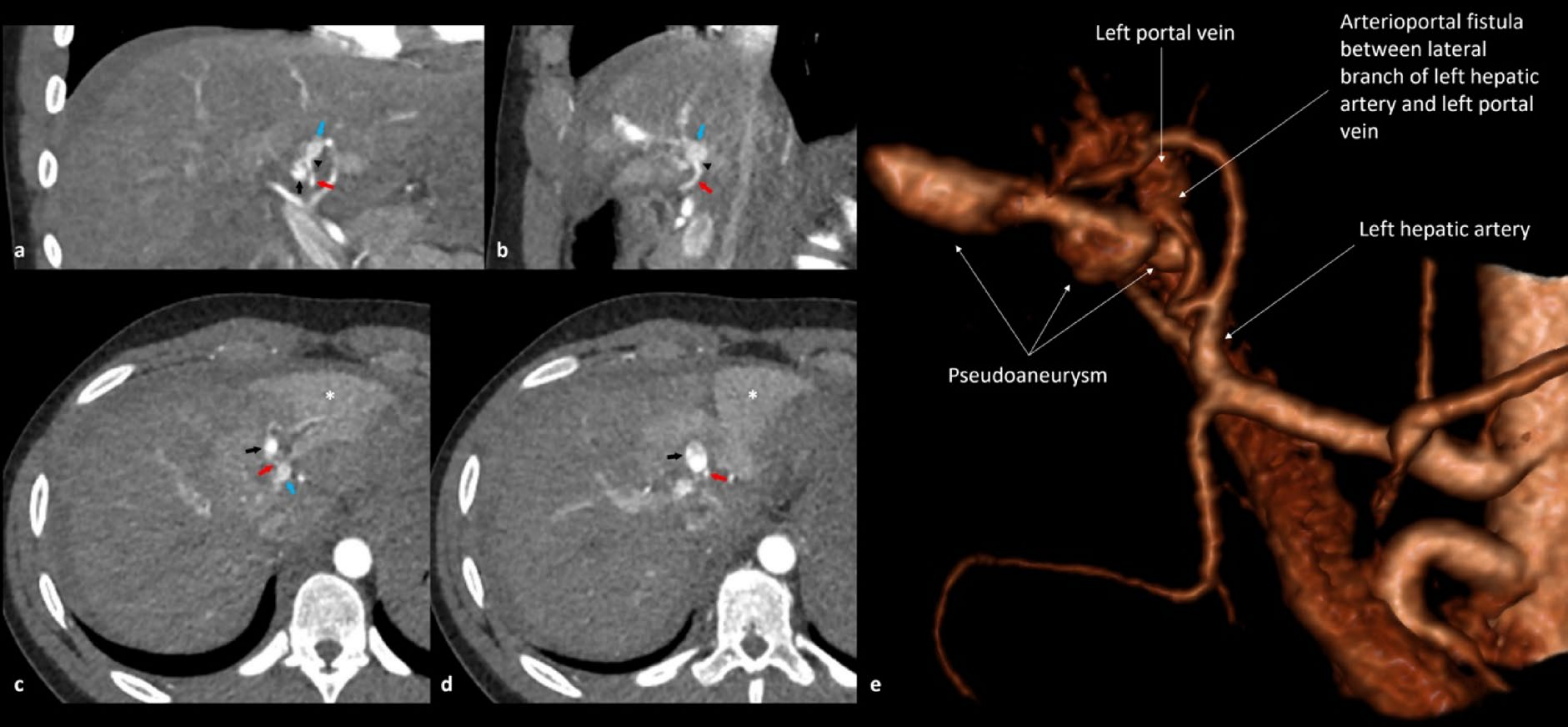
Parasternal right-sided stab wound with injury to the liver. Images a-d show the trauma CT scan in arterial phase in coronal (a), sagittal (b), and axial views (c and d). There is an arterioportal fistula (black arrowhead) between the lateral branch of the left hepatic artery (red arrow) and the left portal vein (blue arrow) with formation of a pseudoaneurysm (black arrow) extending along the stab wound tract in the left liver lobe. Note the arterioportal fistula associated transient hepatic attenuation difference (THAD) of the left liver lobe (white asterisk). e) The image represents the corresponding 3D rendering of the same CT scan. The arterioportal fistula (white arrow), the left portal vein (white arrow), the pseudoaneurysm (white arrows) and the left hepatic artery (white arrow and text) are shown in the image. Non-operative management was applied.
